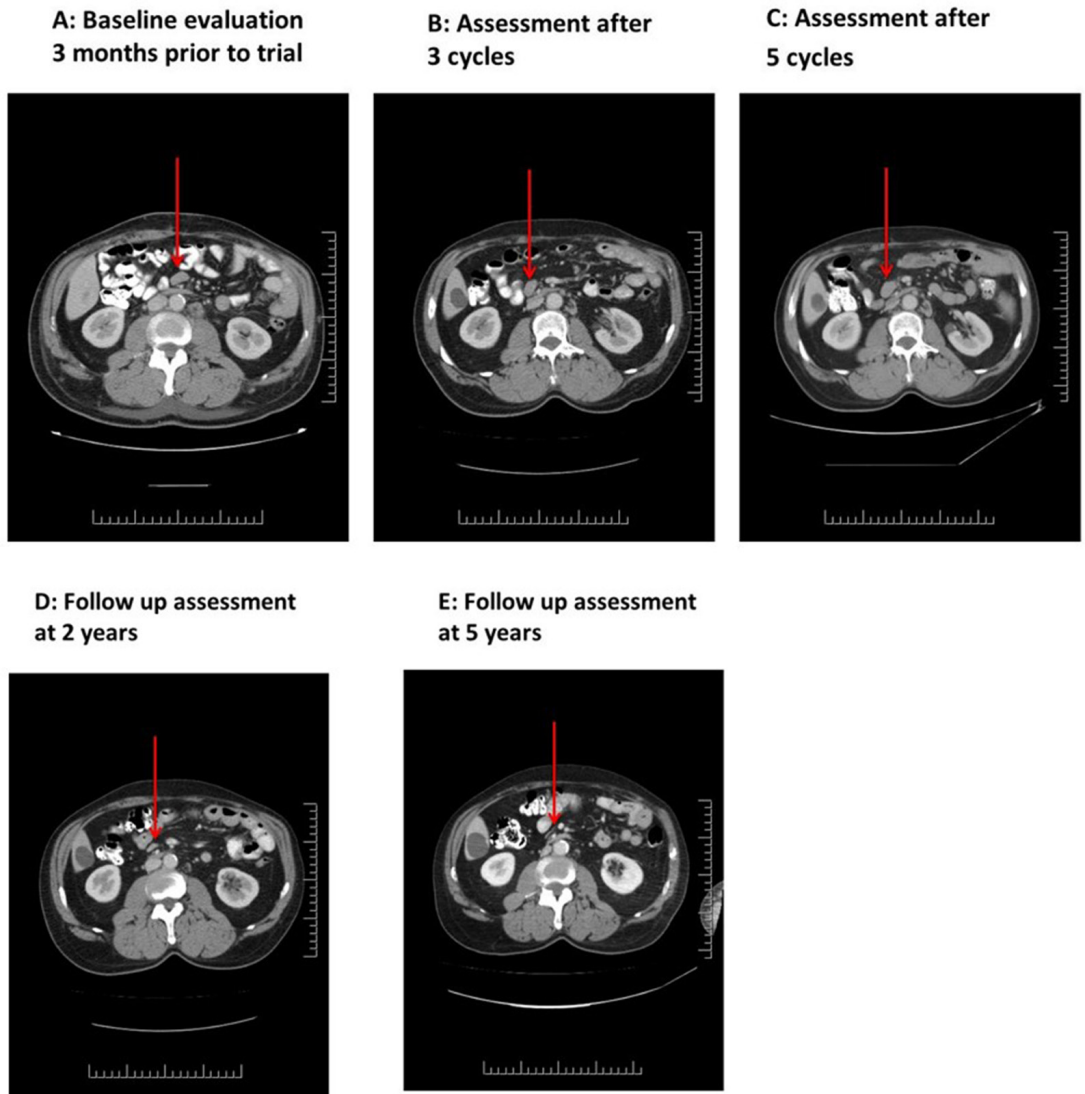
Patient 03 serial CT scans showing appearance of mesenteric lymph nodes prior to NK-92 administration (A) post 3- cycles of NK-92 (B) post 5-cycles of NK-92 therapy (C) and at long term follow-up 2 (D) and 5 (E) years after treatment.
